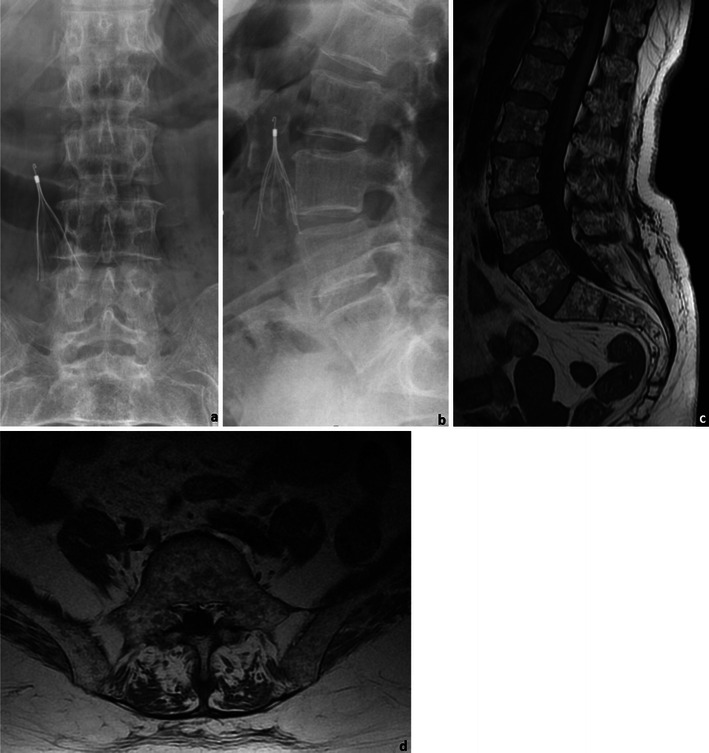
A 76-year-old female patient with MM: Frontal (a) and lateral (b) radiographs of the lumbar spine and sagittal (c) and axial (d) T1WI of the lumbosacral spine. The radiographic study shows diffuse osteopaenia of the lumbar vertebrae, which could only be related to primary osteoporosis given the patients’ gender and age. The MRI study however showed innumerous focal lesions, consistent with the diagnosis of MM. Note: The small cone-shaped device present in the radiographs is an inferior vena cava filter
